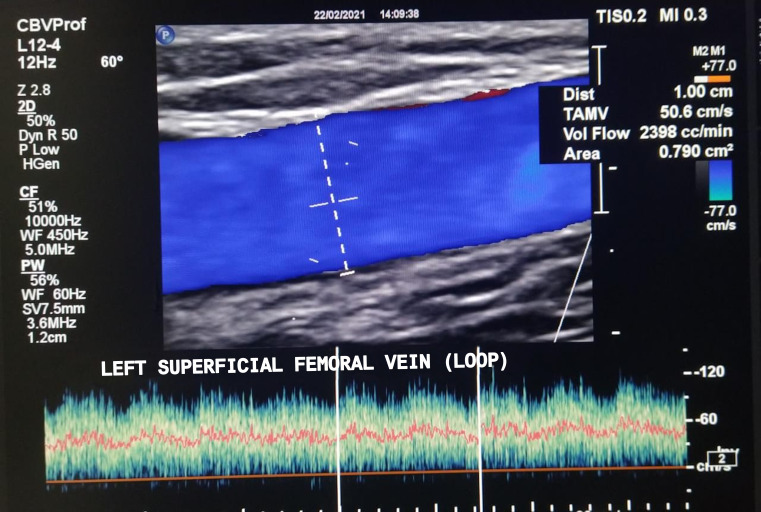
Control Doppler at 3 months. Image showing the 10mm diameter of the superficial femoral vein and flow volume close to 2.4 L/min. Absence of stenosis in the mid venous segment.
